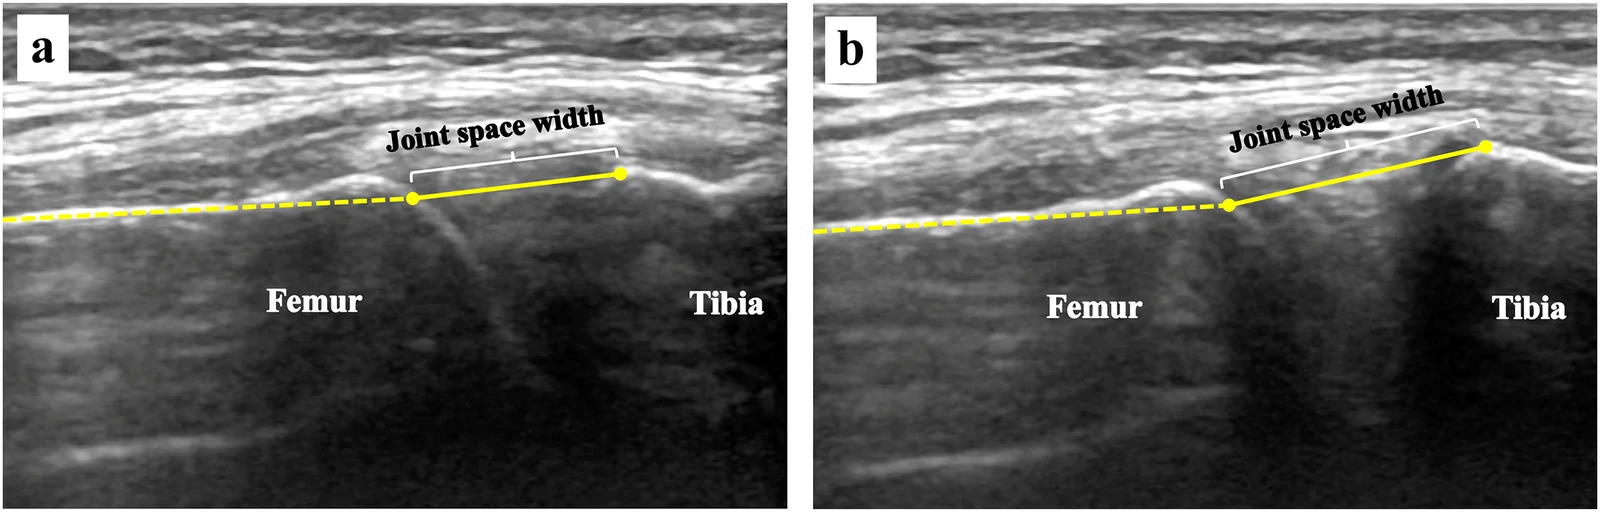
Ultrasound images of the medial knee joint space width. (a) Unloaded condition. (b) Loaded condition. The joint space width was measured as the distance (solid yellow line) between the distal femur end, defined by the extension of the medial femoral margin (dashed yellow line), and the most proximal medial bone surface of the tibial plateau.
